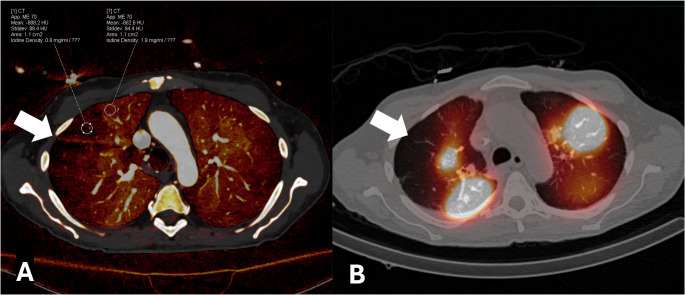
Dual energy CT perfusion blood volume map vs. VQ scan. A 61-year-old man with chronic thromboembolic pulmonary hypertension. A Dual energy CT perfusion blood volume map demonstrates multifocal lobar perfusion defects (white arrows). Quantitative iodine measurements show markedly reduced iodine density within the perfusion defect (0.4 mg/mL) compared with normally perfused lung (2.1 mg/mL). B Same-day V/Q SPECT perfusion imaging in the axial plane demonstrates corresponding perfusion defects in the same regions (white arrows), confirming true perfusion abnormalities
